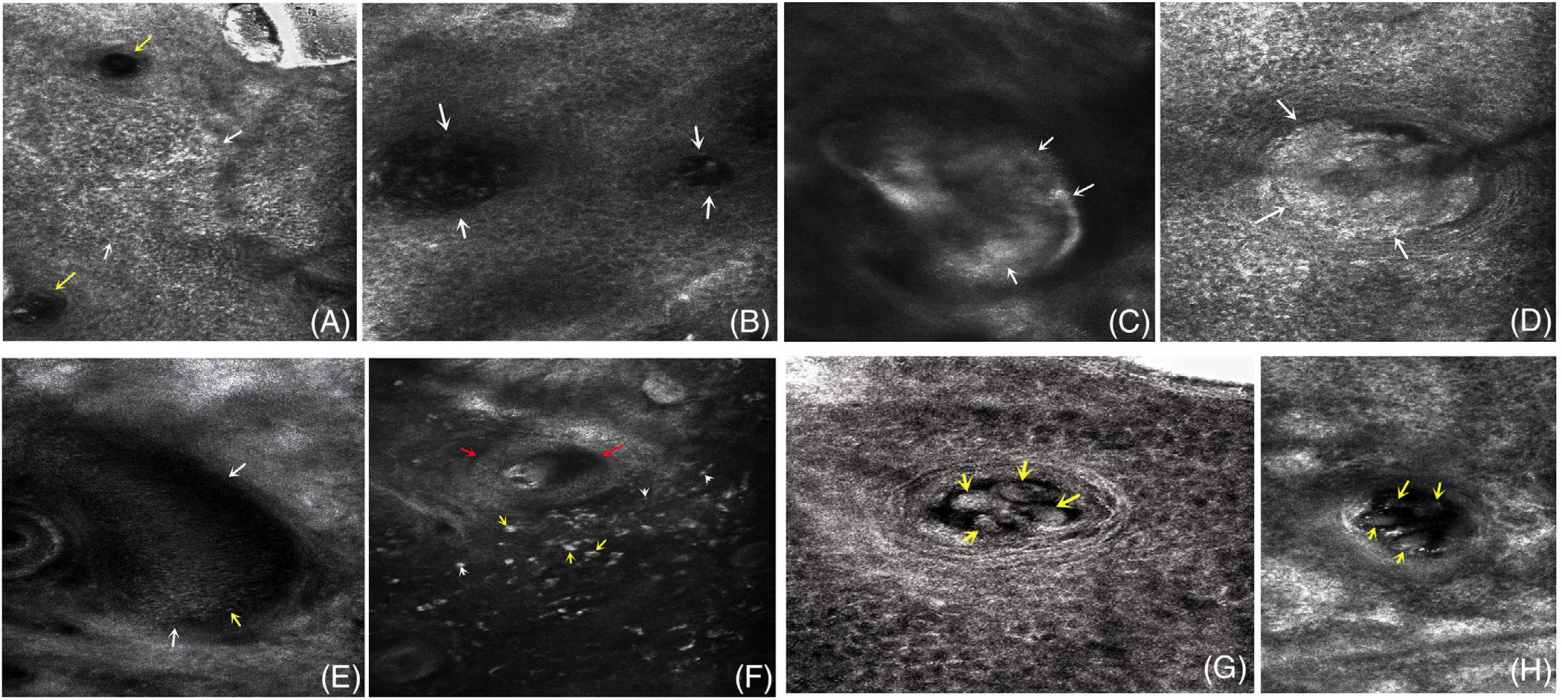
(A) CT scan of the skin showed abrupt changes in the epidermis (white arrow) and hair follicles (yellow arrow); (B) spongy edema in the spinous layer, with low refractive index blisters of different sizes (white arrow); (C) the infundibulum of hair follicle is dilated and filled with high refraction horny like material (white arrow); (D) abscess formation around the hair follicle. High refraction neutrophils can be seen gathering (white arrow) in a vortex shape; (E) the blood vessels around the hair follicle are obviously proliferated and dilated (white arrow), and a large number of low and medium refraction blood cells can be seen (yellow arrow); (F) hair follicle horn plug (red arrow), more inflammatory cells (white arrow) can be seen in the shallow middle layer of dermis and around hair follicles, and some cells are large (yellow arrow); (G) Demodex structure (yellow arrow) can be seen in hair follicle.
